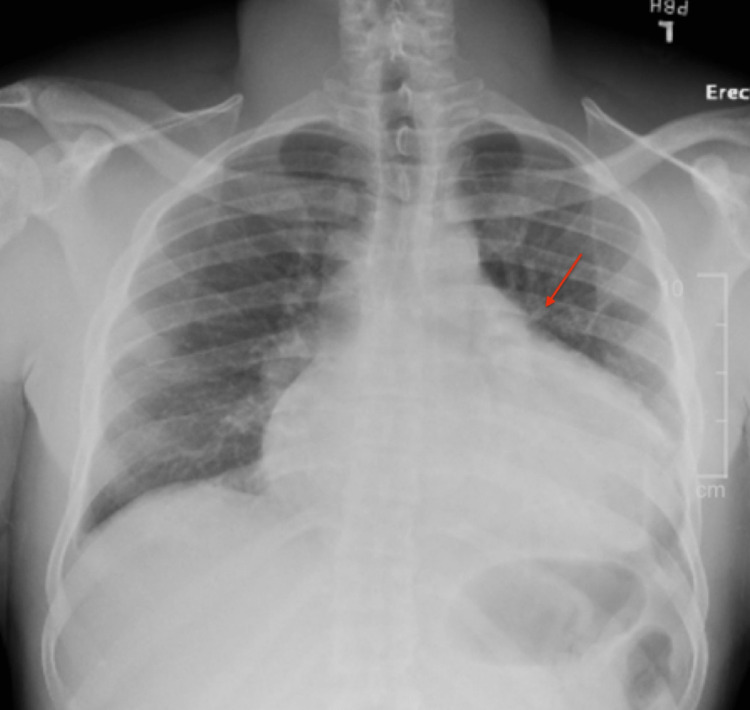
Chest X-ray demonstrating a widened mediastinum.
